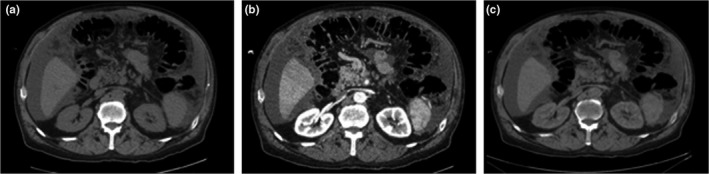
(a) True unenhanced image, (b) postcontrast image, (c) virtual unenhanced image reconstructed from Dual‐Energy computed tomography dataset.
